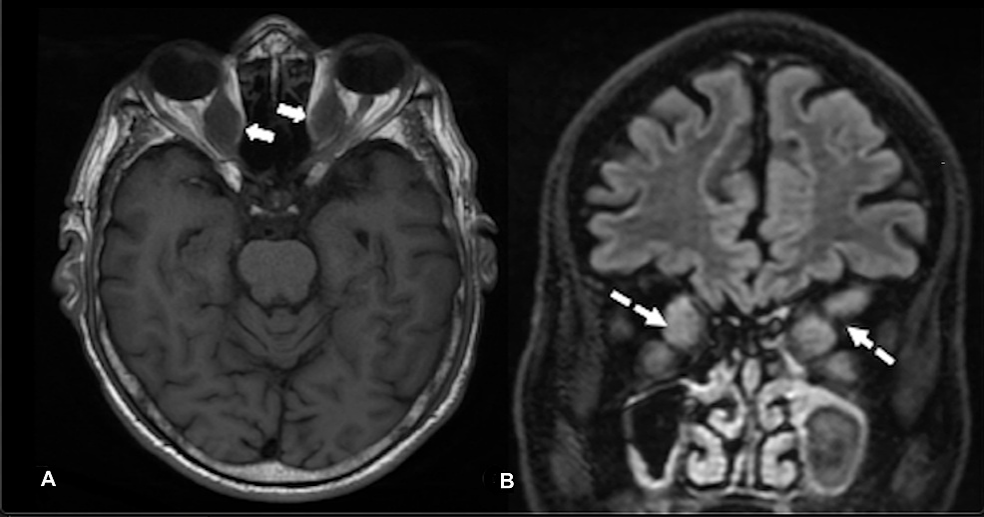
MRI brain demonstrating the 'Coca Cola Bottle' sign. A. MRI brain (axial section) T1 sequence shows symmetrically enlarged medial rectus muscle bellies (closed arrows) with sparing of the tendinous insertions, suggestive of the ‘Coca Cola Bottle’ sign. B. MRI brain (coronal section) T2/FLAIR sequence shows enlarged medial, superior, and inferior rectus muscle bellies (dashes arrows).
